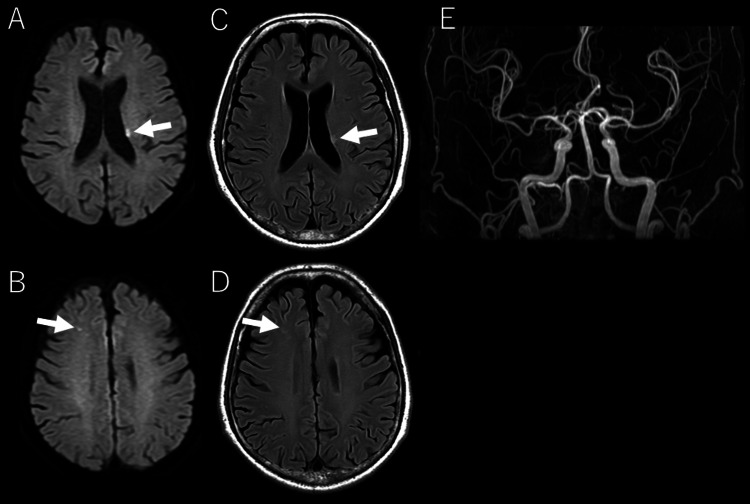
Head MRI and MRA. DWI (A, B) and FLAIR (C, D) show high signal areas in the left corona radiata and frontal lobe, consistent with acute to subacute ischemic infarction. MRA (E) reveals no significant aneurysms. DWI, diffusion-weighted imaging; FLAIR, fluid-attenuated inversion recovery; MRI, magnetic resonance imaging; MRA, magnetic resonance angiography
